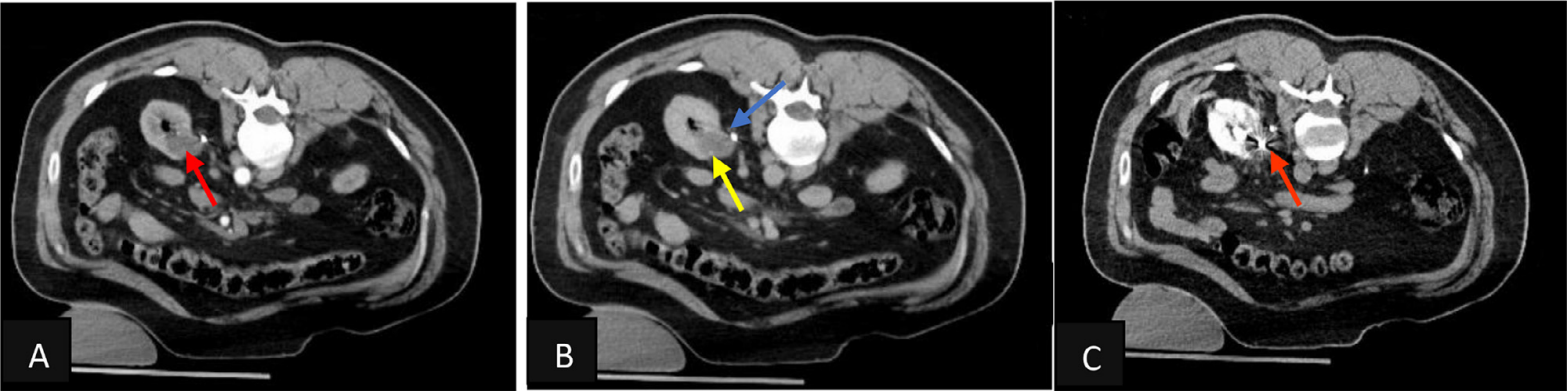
Triphasic CT scan during cryoablation procedure (A) showing the tumor in anterior lower calyx of left kidney in arterial phase (red arrow), (B) delayed excretory phase showing the close proximity of the tumor (yellow arrow) to the ureter (blue arrow), (C) the intraprocedural CT scan showing cryoablation of the tumor with possible involvement of the upper ureter (orange arrow).
